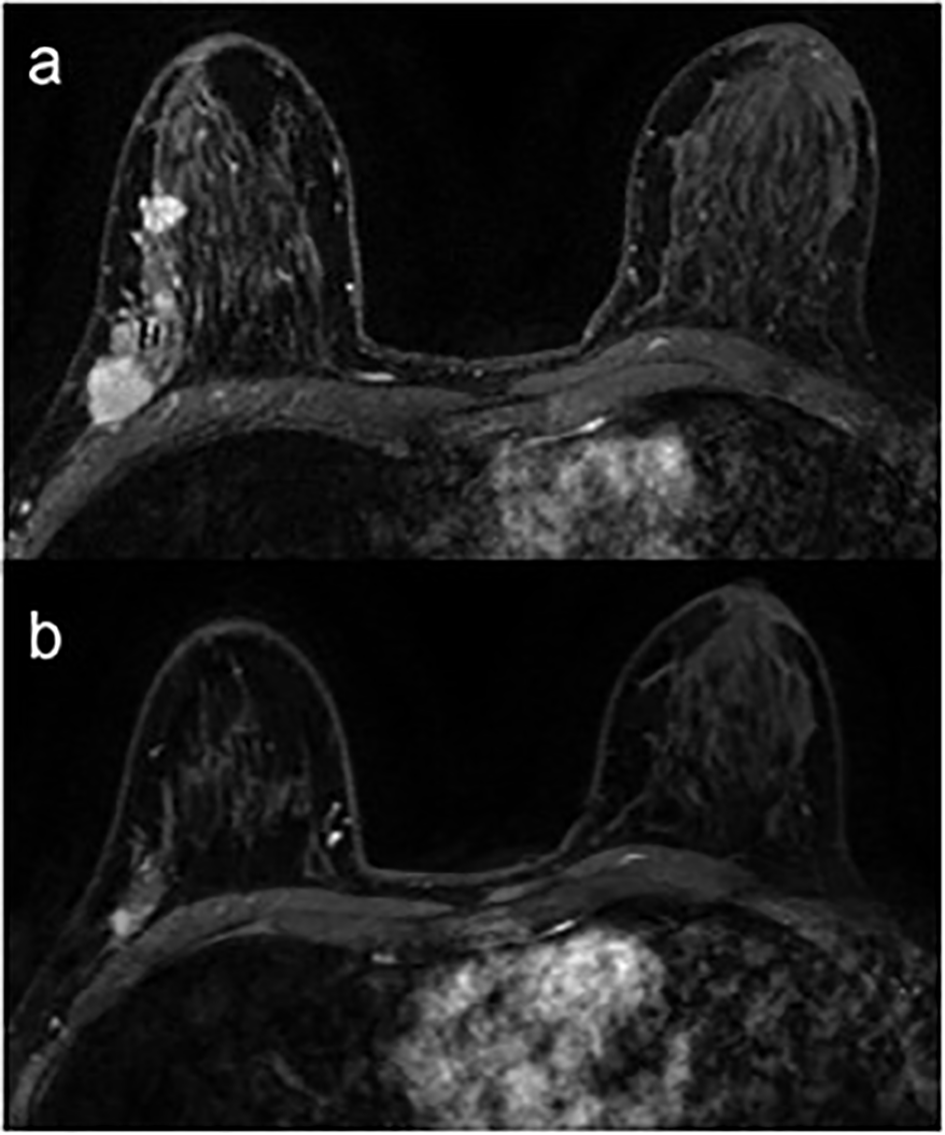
Breast MRI of a 53-year-old obese woman (VAT = 152.8 cm^2^; VAT > 130 cm^2^ = visceral obesity) with a multifocal breast cancer of the right breast. Axial post-contrast T1-weighted images before (A) and after (B) 6 months of CDK 4/6 inhibitors show a good response to treatment, as documented by the reduction in size of all lesions in the upper-outer quadrant/axillary extension of the right breast.
